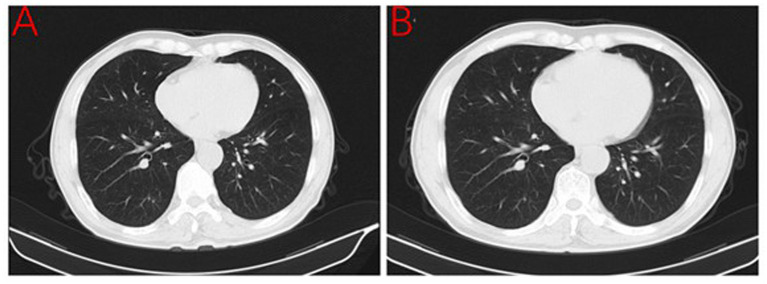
Follow-up chest CT findings. (A) At 3 months after discharge and (B) at 9 months after discharge: marked improvement compared with the initial admission CT findings.
